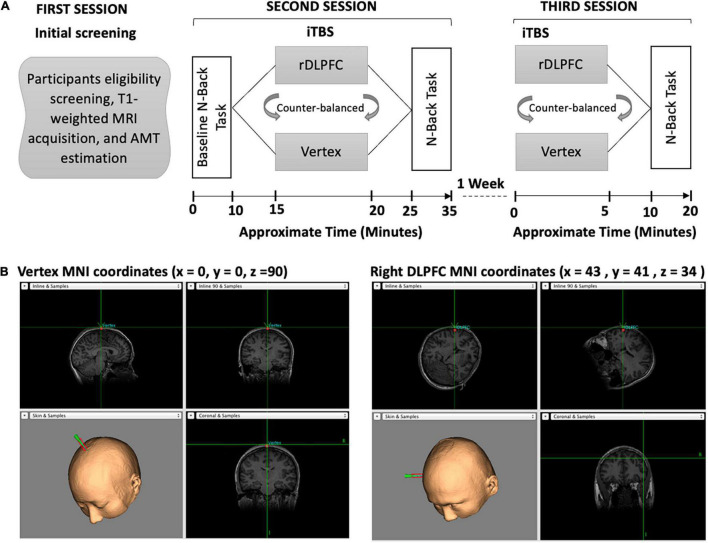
(A) Overview of the experimental paradigm. Our experiment consisted of three separate sessions. During the first session, the participants were screened on eligibility, had their T1-weighted images acquired, and AMT estimated. In the second session, participants performed the baseline n-back task followed by iTBS and then the post-stimulation n-back task. In the third session, the participants received iTBS and performed the post-stimulation n-back task. (B) The iTBS target brain areas, the vertex and the Rdlpfc, respectively.
